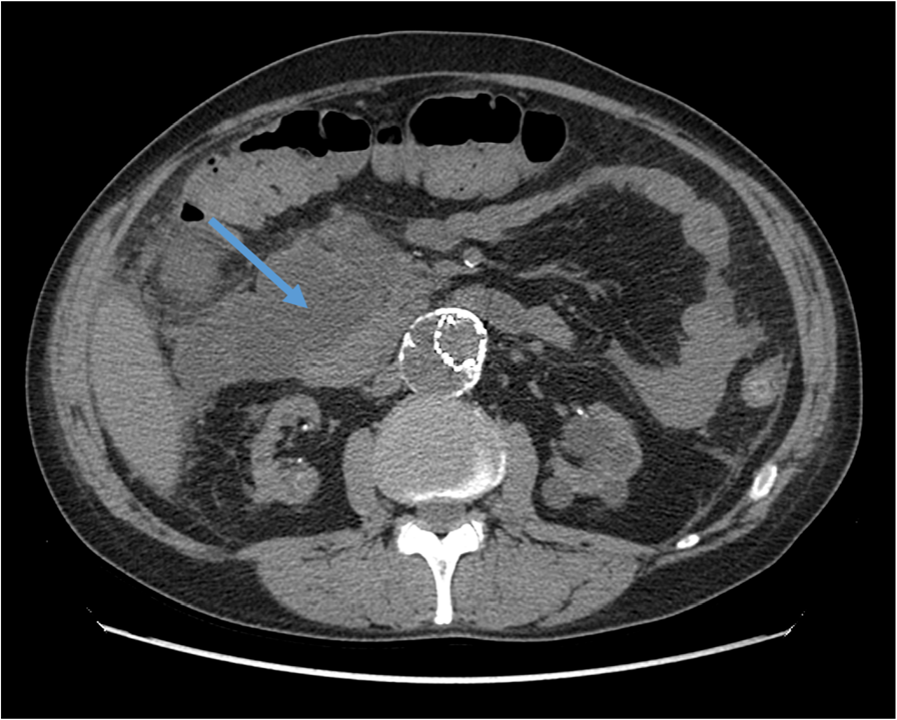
Abdominal CT Scan showing the initial area of patchy necrosis around the head of pancreas
